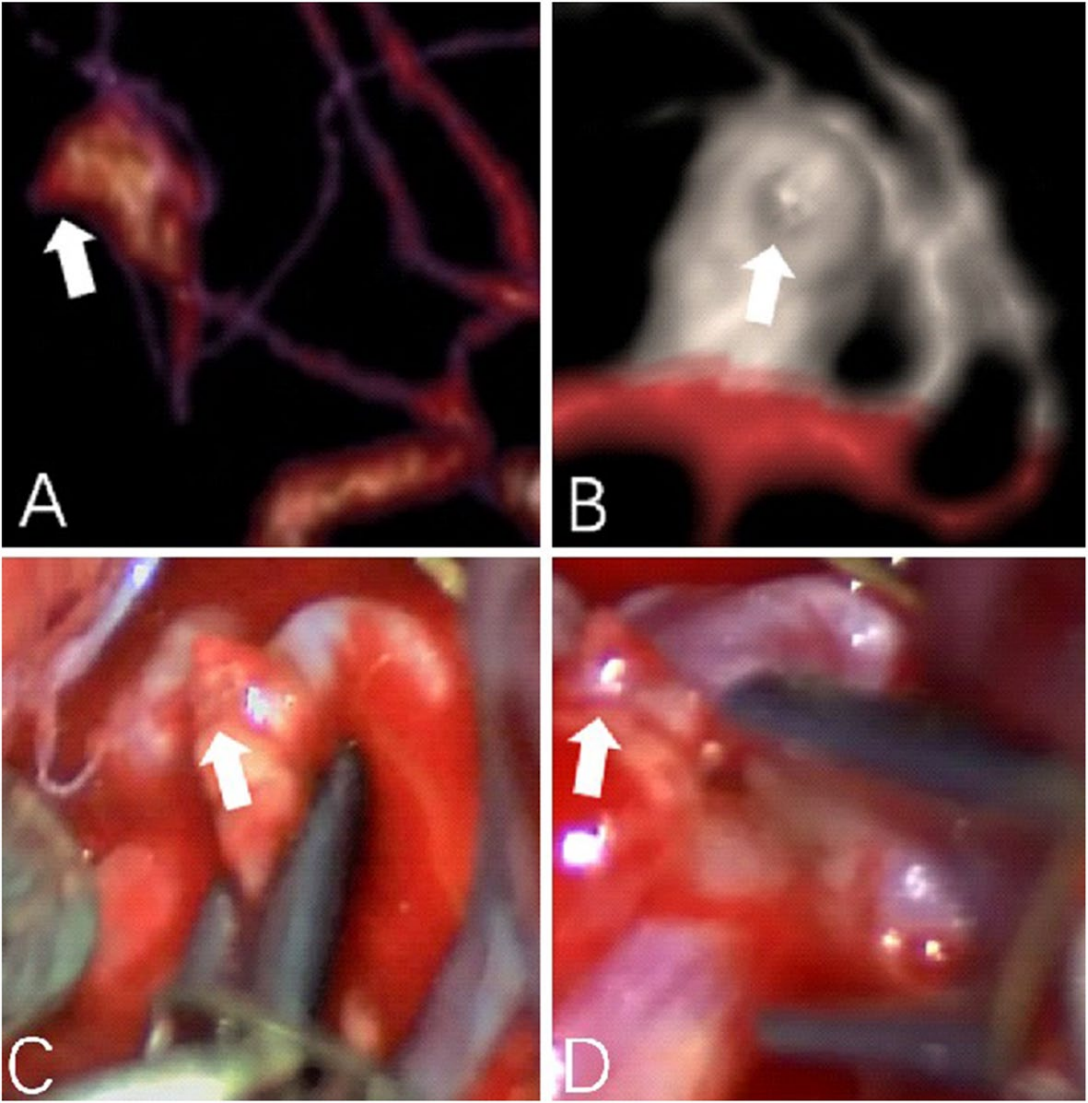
A-B CTA showed a right MCA-M1 aneurysm (7.6 mm x 5.1 mm), and a tiny pulsation was observed by 4D-CTA (indicated by the white arrow. C-D photographs obtained during surgery, small vesicles shaped convex were seen intra-operation (as indicated by the white arrow), intra-operational findings confirmed that the abnormal pulsation site is an at-risk aneurysmal wall
